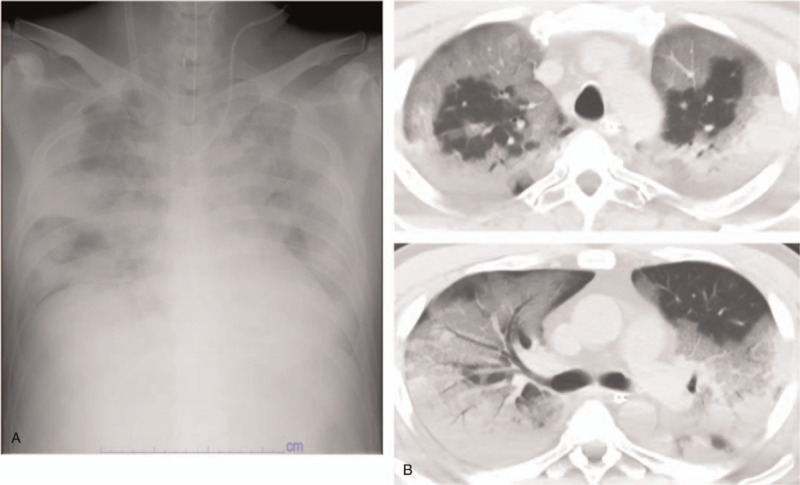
Left panel (A), bilateral alveolar infiltrates on chest x ray with normal cardiac size consistent with ARDS; right panel (B), a series of high resolution chest CT scans demonstrating bilateral extensive ground glass opacities. ARDS = acute respiratory distress syndrome.
